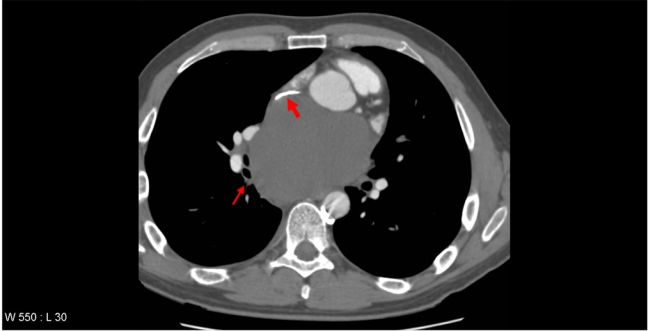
Computed tomography imaging of chest, with intravenous contrast showing superior vena cava compression (small arrow) and right inferior pulmonary vein compression and thrombus (large arrow).
